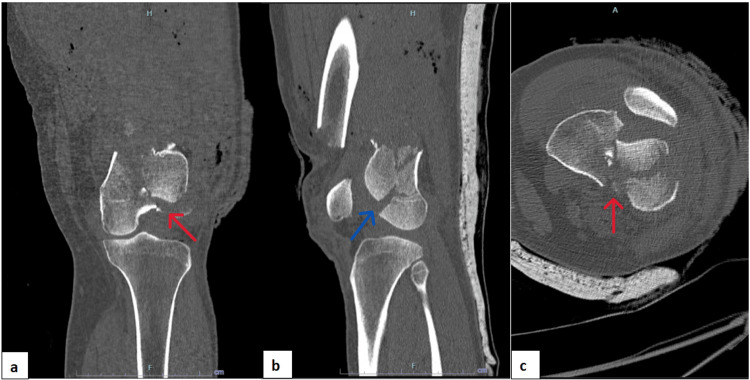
Computed tomography scan of the left knee showing a comminuted fracture of the left distal femur with an intercondylar split and Hoffa fragment at the lateral femoral condyle. (a) Coronal view. (b) Sagittal view. (c) Axial view. The red arrows show intercondylar split, and the blue arrow shows the Hoffa fragment.
